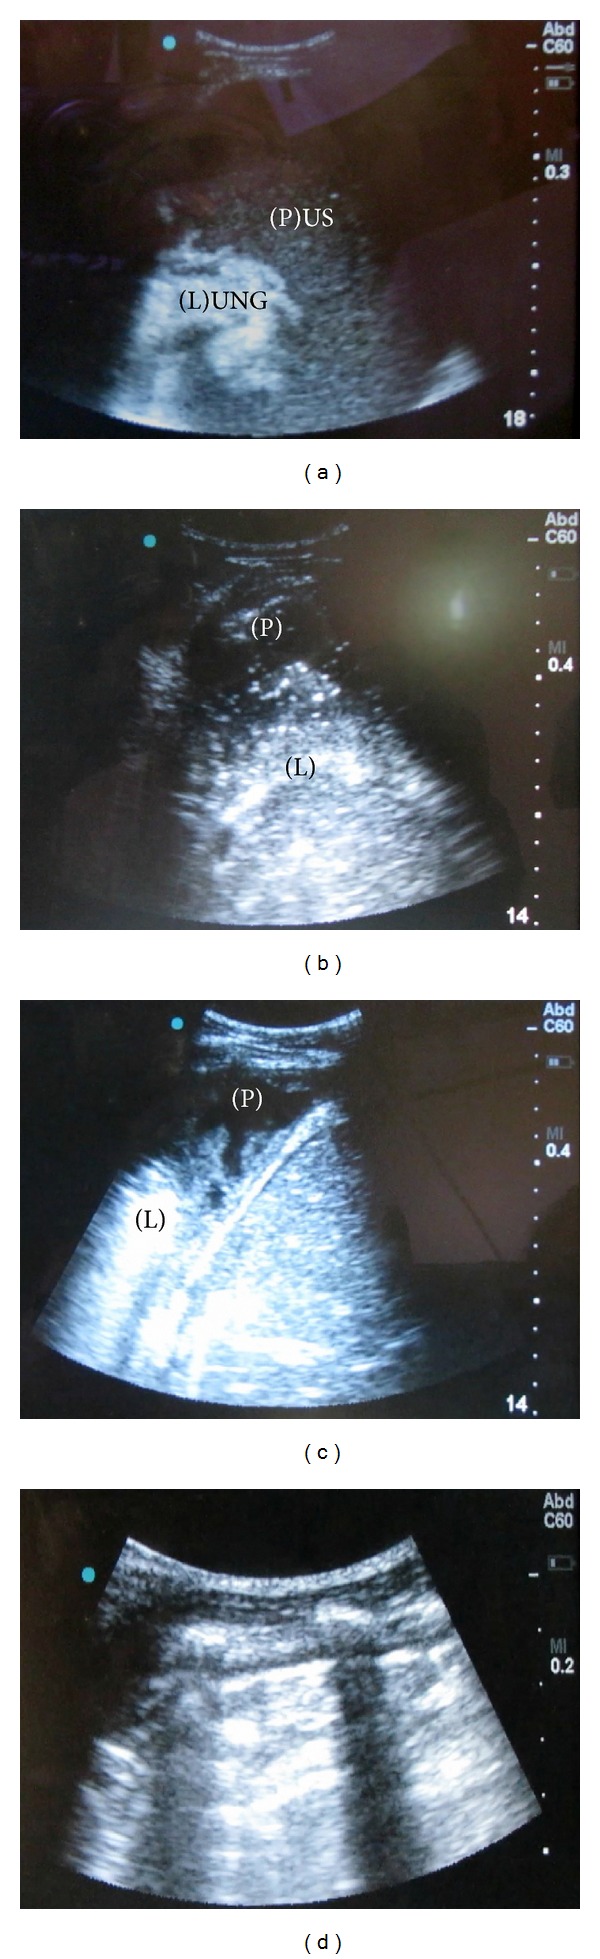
(a) The initial ultrasound demonstrating a large amount of fluid in the pleural space and a collapsed lung. (b) An ultrasound one day after the drain was placed demonstrating decreased fluid in the pleural space. (c) An ultrasound three days after the drain was placed demonstrating even less fluid and improved lung expansion. (d) An absent sliding sign on the affected hemithorax.
